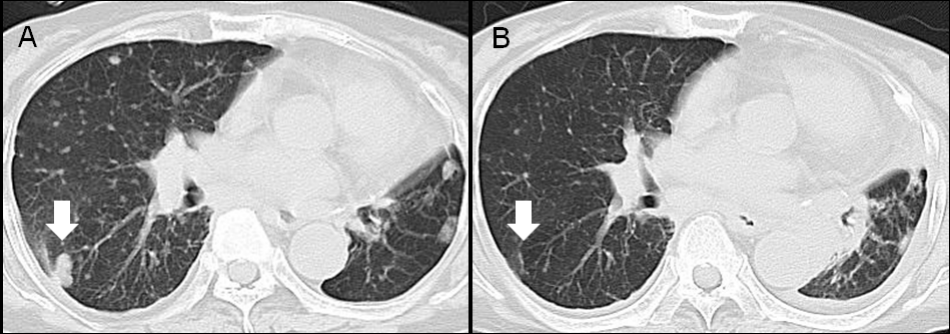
A case of AXL 3+-expressing lung adenocarcinoma that responded well to gefitinib. (A) Pre-treatment and (B) post-treatment (6 months after initiation of gefitinib, CT scans are shown.
